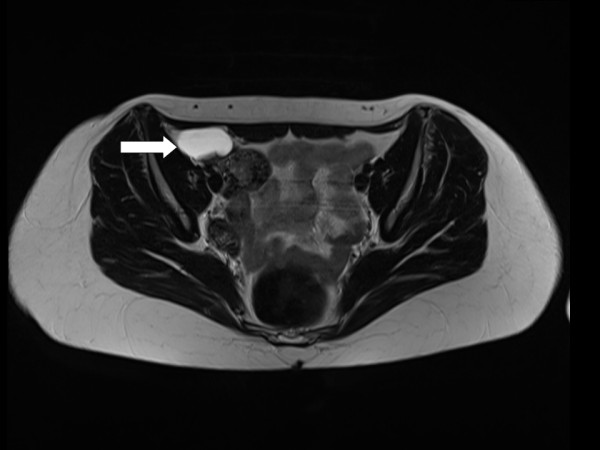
MRI scan showing the cystic lesion in the cecal region (arrow).
